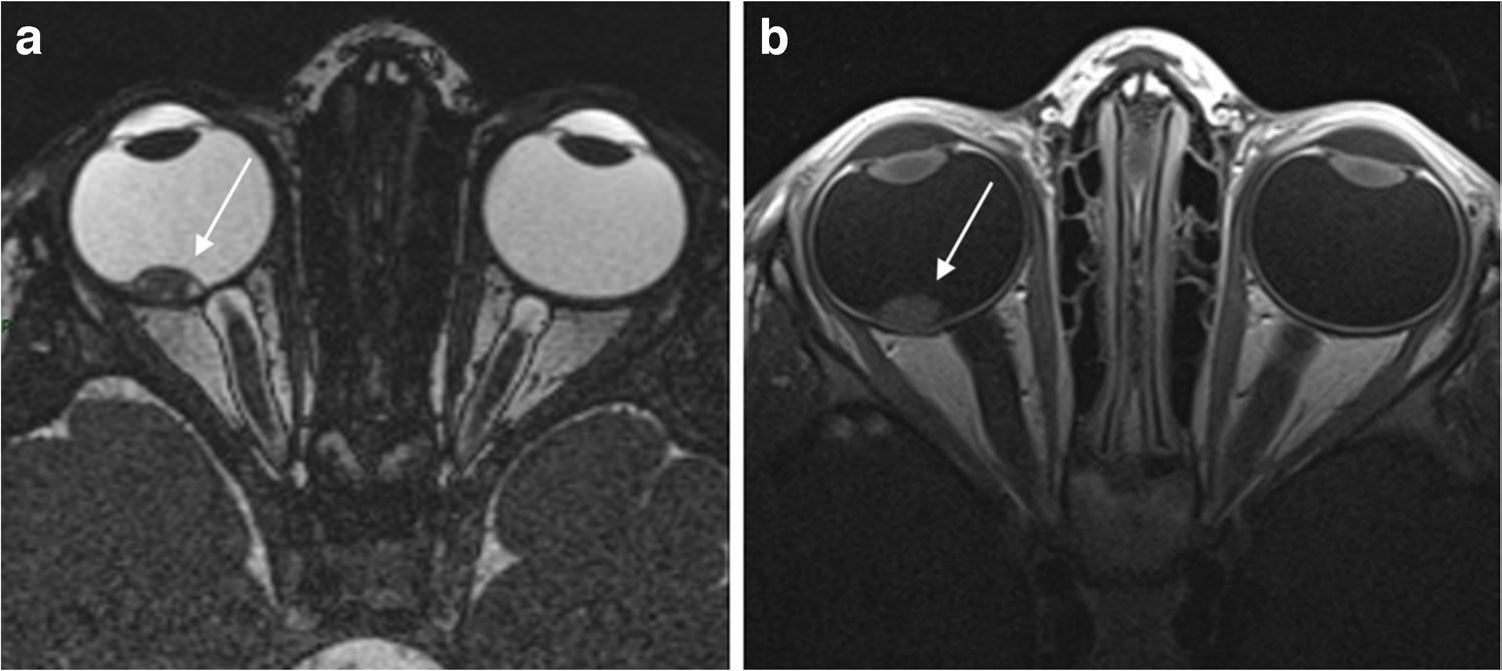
MRI of a 30-month-old girl at the time of imaging, previously diagnosed with retinocytoma. Axial T2-weighted (a) and post-contrast T1-weighted (b) images show (*arrow*) an increase in size and degree of contrast enhancement of the macular mass compared with prior imaging. *MRI*, magnetic resonance imaging
